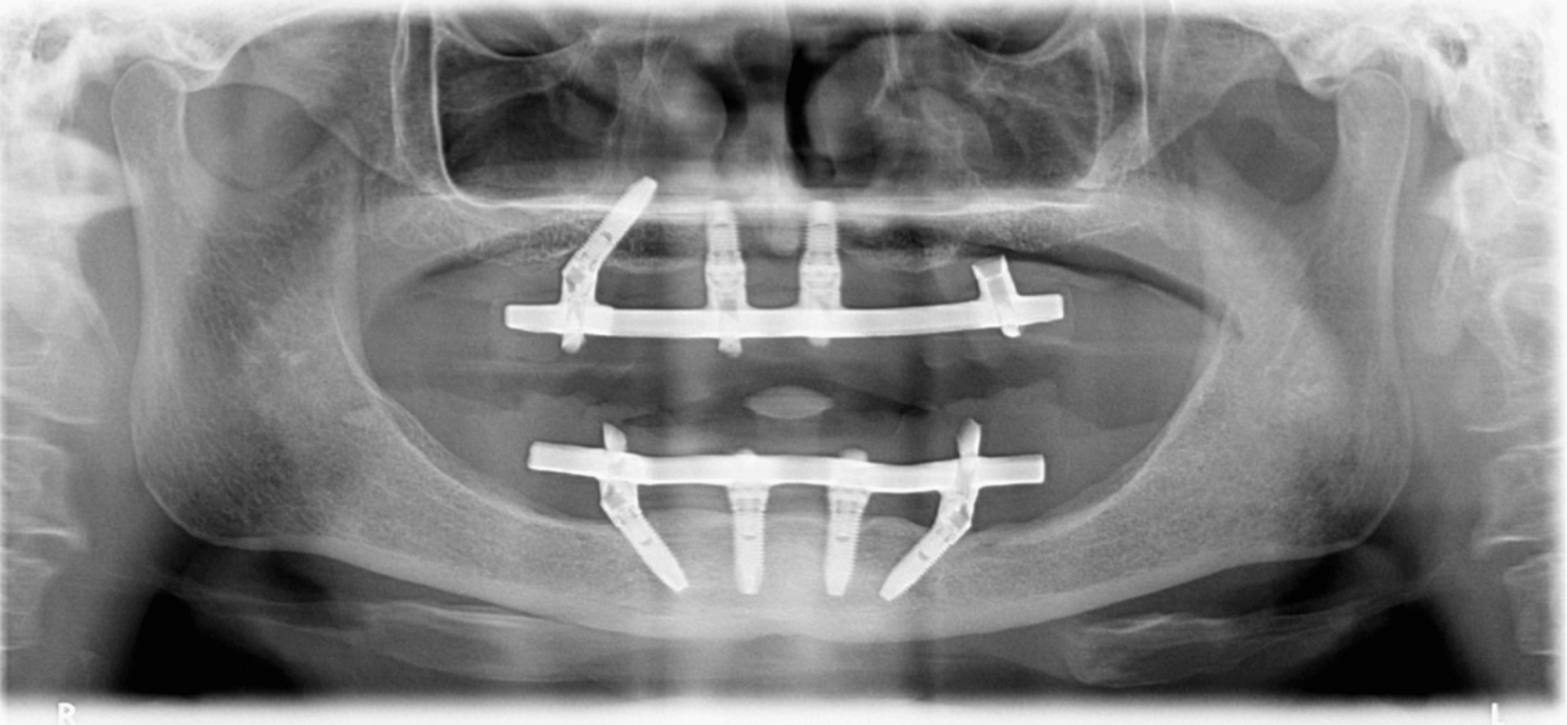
A preoperative orthopantomogram of the maxilla, indicating the absence of a posterior tilted implant in the third sextant. Reproduced without modification from reference [41] under the Creative Commons License (CC BY 4.0). For further details, please visit the Creative Commons License (http://creativecommons.org/licenses/by/4.0/).
